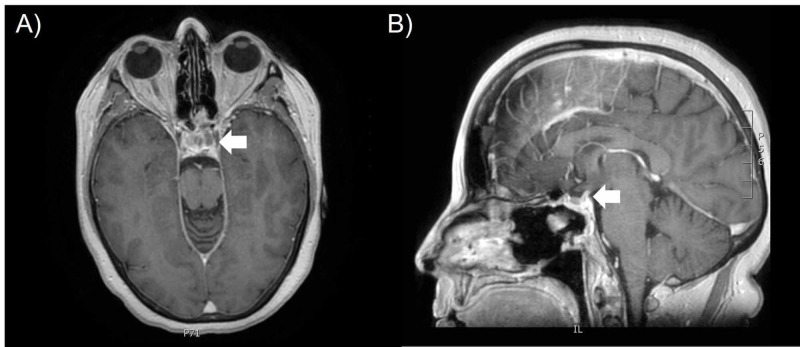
MRI T1-FLAIR images of the pituitary tumor after transsphenoidal resection and prior to radiation treatment. The patient underwent transsphenoidal resection of the anterior wall, sphenoid sinus, posterior nasal septum, posterior ethmoid sinuses prior to stereotactic radiotherapy. There is some low signal within the sella on the left side that may represent residual tumor (arrow). No mass is seen in the suprasellar region or cavernous sinus. (A) Axial view; (B) Sagittal view.
